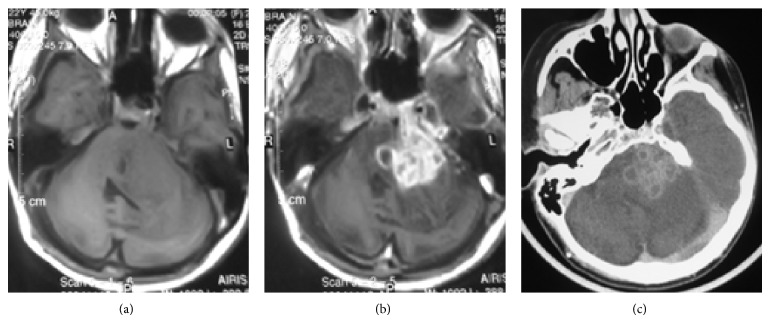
Tuberculous abscess mimicking a cerebellopontine angle tumor in a 22-year-old female with miliary pulmonary tuberculosis, right hemiparesis, left facial paresis, and sixth and seventh cranial nerves involvement. Axial T1-weighted image (a) shows a predominantly isosignal lesion in the left hemisphere of cerebellum with extension to CPA and prepontine cistern accompanied by marked peripheral edema and mass effect. Multilobulated enhancement of the lesion is seen in the postcontrast T1-weighted (b) and CT (c) images.
